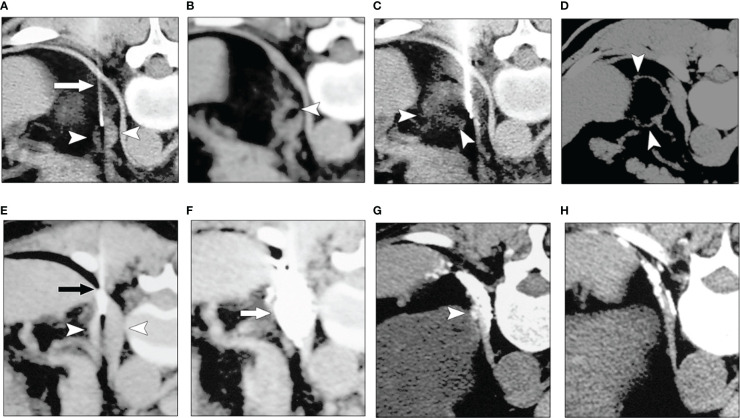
Chemical ablation of left adrenal adenoma with anhydrous alcohol (A–D). (A) Transverse CT image obtained with the patient in a prone position showed a fine needle (arrow) in the left adrenal mass (arrowheads). (B), CT image obtained after the first injection of alcohol revealed a focal collection of alcohol in the adrenal tumor (arrowhead). (C), CT image obtained after the third ethanol injection shows the distribution of alcohol in tumor (arrowheads) was more diffuse than in (B, D) Follow-up CT image obtained one year after (A–C) revealed that most of the lesion (arrowheads) was replaced by fatty tissue. Chemical ablation for left adrenal metastases from the renal carcinoma after total left nephrectomy (E–H). (E) A transverse CT scan with the patient in a prone position showed a needle (arrow) in the left adrenal mass (arrowheads). (F) The image obtained after the second injection of acetic acid and iodized oil showed good compound permeation (arrow). (G) Six months later, the follow-up CT image showed a remaining dotlike lesion (arrowhead), and iodized oil was within the diaphragm. (H) One year after A and B, a follow-up CT image showed the lesion had fully resolved (4). (Zhang JS et al, *AJR*, 2008).
